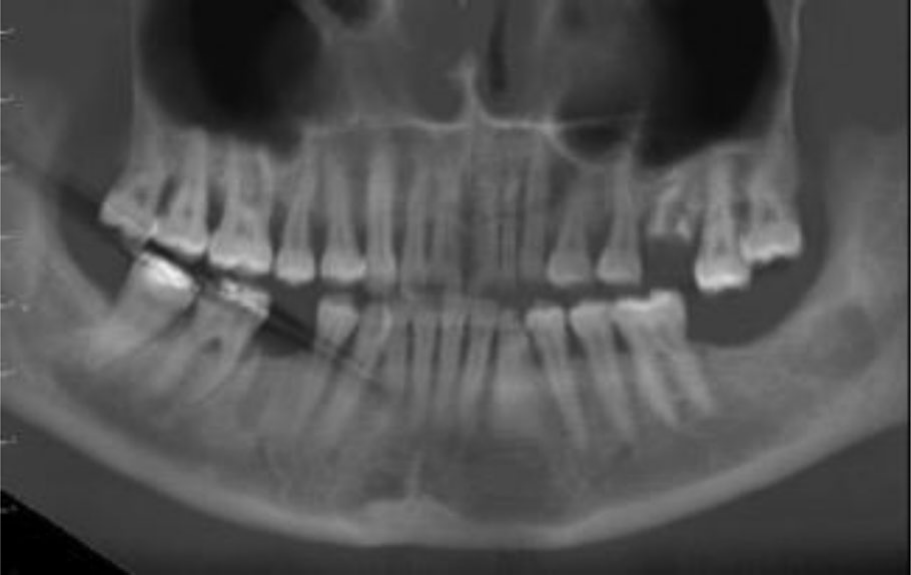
Panoramic reconstructed in thick section from a CT scan “head bone” volume.
